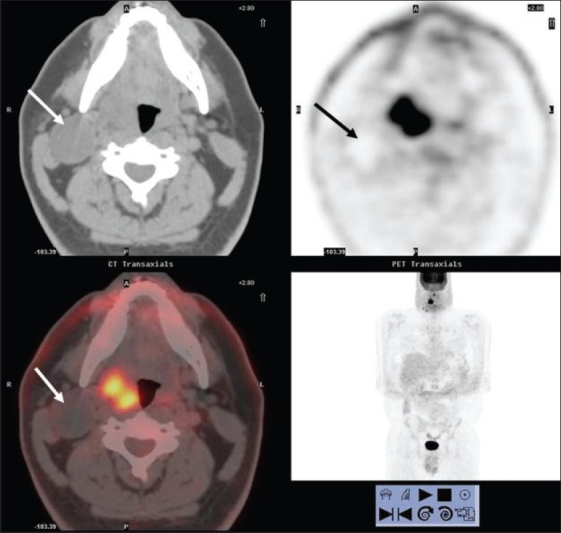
Axial PET (top right), CT scan (top left) and fused PET/CT (bottom left) images at the submandibular region level, with an MIP image (bottom right), from a PET study of a 44-year-old man with right tonsillar cancer that is intensely FDG avid. However, an enlarged hypodense nodal mass (white arrows) posterior to the angle of the right mandible is completely ‘cold’ on PET but clinically was hard and consistent with metastasis (also confirmed on subsequent neck dissection)
